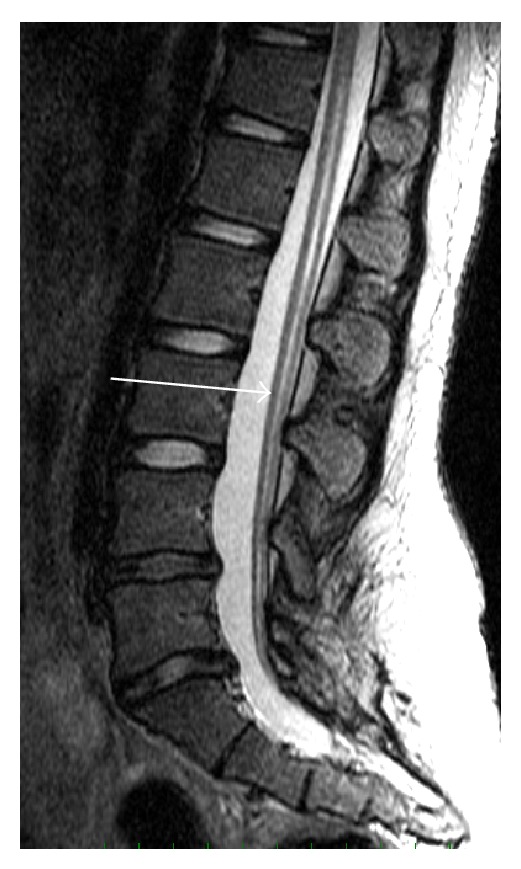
Sagittal T2-weighted magnetic resonance imaging showed a tethering cord with a fatty filum terminale (white arrow).
